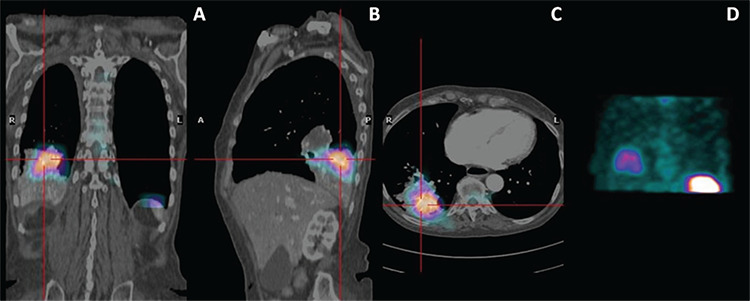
Fused technetium-99m (Tc-99m)-hexamethylpropylene amine oxime (HMPAO)-labeled leukocytes single-photon emission computed tomography/CT (SPECT/CT) hybrid images on coronal (A), sagittal (B), and transaxial (C) planes with Tc-99m-HMPAO-labeled leukocytes chest SPECT coronal maximum intensity projection (D). Principal clinical indications for Tc-99m-HMPAO-labeled leukocytes scintigraphy include inflammatory bowel disease, osteomyelitis, soft tissue sepsis, and occult fever (1,2). Autologous leukocytes are characterized by high specificity because they only accumulate into the inflamed tissues due to active migration and because very infrequent fixation occurs in neoplastic tissues (1,3). A 71-year-old Caucasian female was admitted to our department for an intermittent fever of unknown origin associated with chill at night for the last month. She was recently diagnosed with bronchopulmonary neuroendocrine tumor at the right lung superior segment of the inferior lobe. A phlebitic process of intravenous catheter was also depicted. Chest CT scan showed a thrombotic widespread of the superior vena cava (SVC) and another one near the infusion catheter localized in the proximal tract of the brachiocefalic artery. Moreover, in the superior segment of the inferior lobe of the right lung, a conglobated solid pathological tissue (50x41 mm^2^) causing thickening and infiltration of the scissural pleura and incorporating the segmental bronchial vessels, particularly the bronchial branches of the postero-basal segment, almost completely obliterated with consensual atelectasis of the segment was reported. Being a carrier of a port-a-cath with the catheter guided into the SVC, an infection of this device or the thrombus described above was suspected. Transthoracic and transesophageal echocardiogram were negative for the infectious source on the SVC catheter and tested blood cultures of all microorganisms. Tc-99m-HMPAO-labeled leukocytes scintigraphy was performed in order to identify the source of infection responsible for her clinical course. Whole body planar and SPECT images of the chest region were acquired. Matching pairs of CT scan and Tc-99m-HMPAO-labeled white blood cell SPECT images were fused using dedicated Xeleris software (GE Healthcare) to generate hybrid images of overlying transmission and emission data. The three-plane triangulation showed the clear match between the densitometric alteration found on CT scan and the markedly pathologic Tc-99m-HMPAO-labeled leukocytes uptake in the inferior lobe of the right lung, thus unveiling the presence of an active infectious disease.
